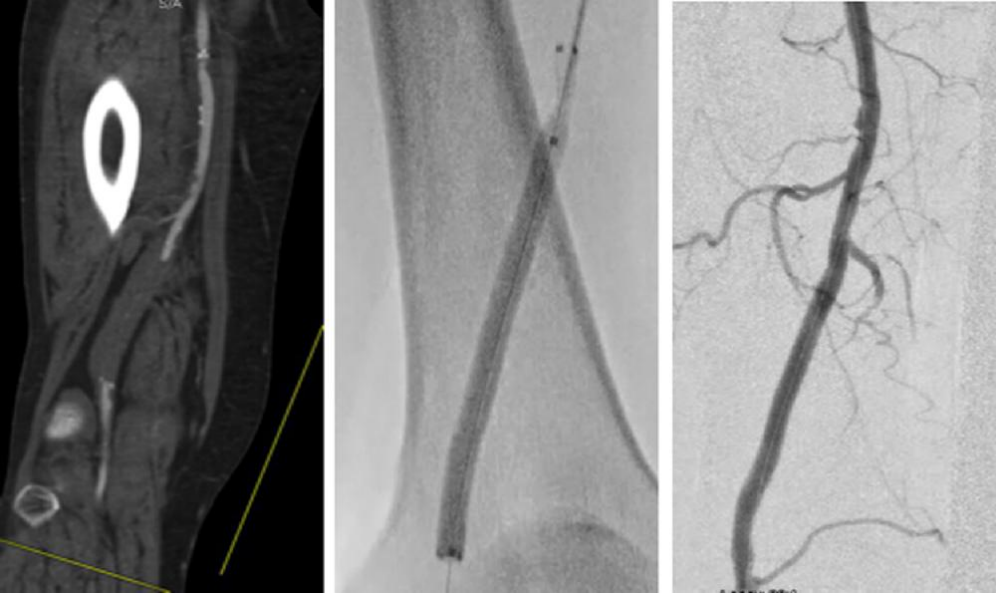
Computed tomography angiography (left panel) and digital subtraction angiography (middle and right panels) images obtained in a patient with intermittent claudication presenting an occlusion of the distal third of the superficial femoral artery, successfully treated with balloon angioplasty.
